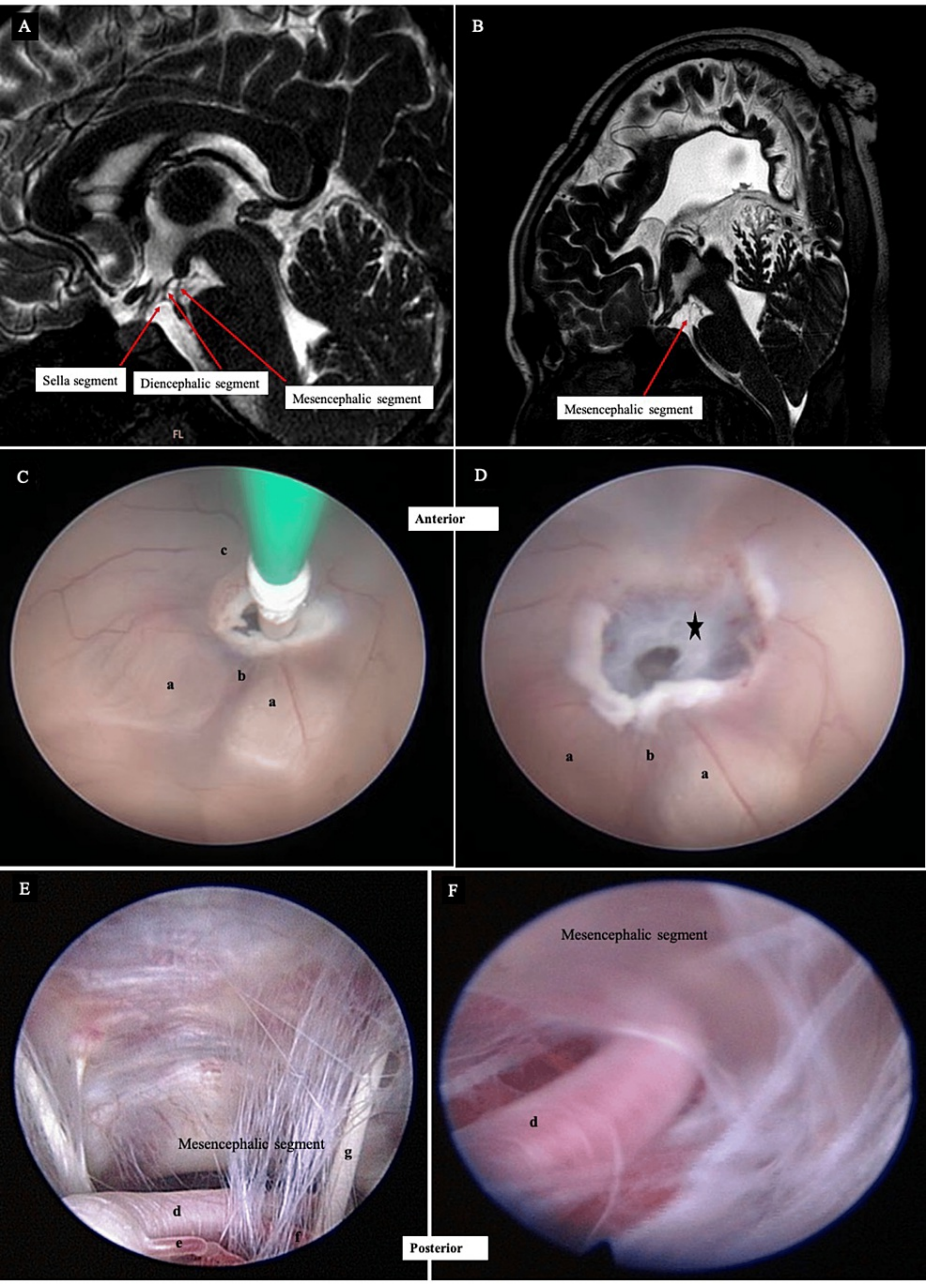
Anatomical images of the Liliequist membrane. (A) Mid-sagittal CSF DRIVE brain MRI of a child shows the segments of the Liliequist membrane. (B) A similar sequence of different children with agenesis of corpus callosum who only have the mesencephalic segment. (C) Neuroendoscopic images were captured during the endoscopic third ventriculostomy procedure in a child with meningitic hydrocephalus. The diencephalic leaf of the Liliequist’s membrane was initially expected to be opened simultaneously with the third ventricular floor during stoma creation. (D) The leaf is found to be a dense sheet-like membrane without openings (star) and requires further coagulation to open the membrane. (E, F) The mesencephalic leaf with variable arachnoid trabeculae and is attached to the oculomotor nerve. a, mammillary bodies; b, basilar artery underneath; c, floor of the third ventricle; d, basilar artery; e and f, perforating basilar artery; g, right oculomotor nerve.
